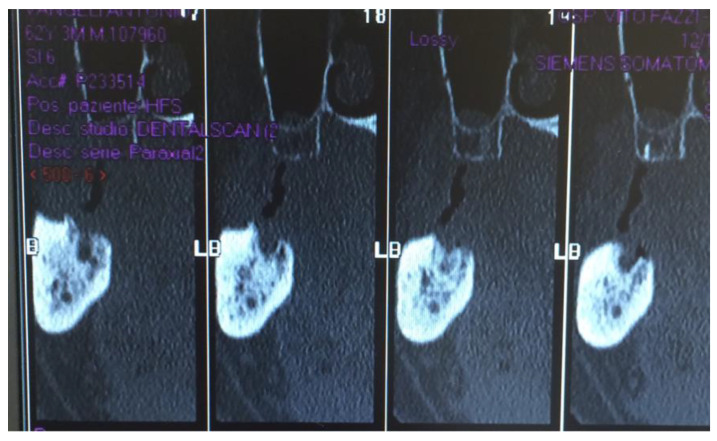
Pre-operative CBCT of the MRONJ patient, showing a reduction in the medullar space.
